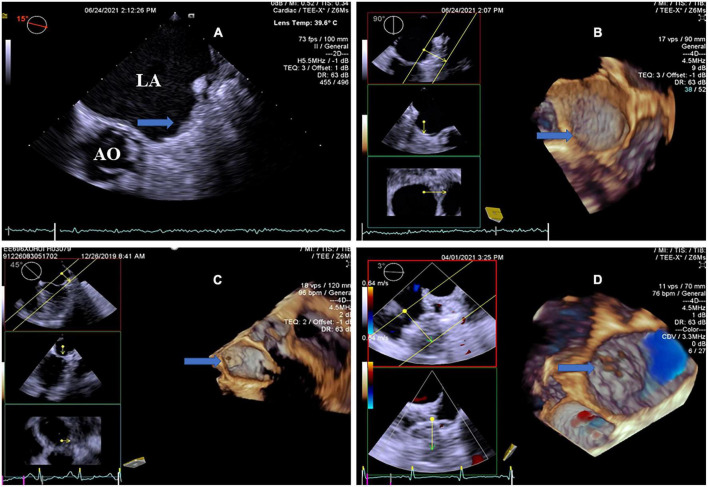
Transesophageal echocardiography follow-up images after LAAC showing optimal device placement and device-related thrombus. (A) 2D-TEE showed an echo-dense mass attached to the LACbes device, occupying most area of the occluder (arrow). Note severe spontaneous echo contrast throughout the left atrium. (B) RT4D-TEE demonstrated a thin layer of thrombi covering the whole surface of the well-seated LACbes device (arrow) after LAAC, as compared with 2D-TEE. (C) RT4D-TEE indicated complete LAA closure with the Watchman device, meanwhile demonstrating a mobile thrombus pedunculated with a neck attached to the occluder (arrow). (D) RT4D-TEE detected optimal device placement and a couple of scattered clots on the surface of the Watchman occluder (arrow). There was no residual peri-device leak at the LAA ostium detected by color Doppler. RT4D-TEE: Real-time four-dimensional echocardiography. LAAC, left atrial appendage closure; 2D-TEE, two-dimensional transesophageal echocardiography.
